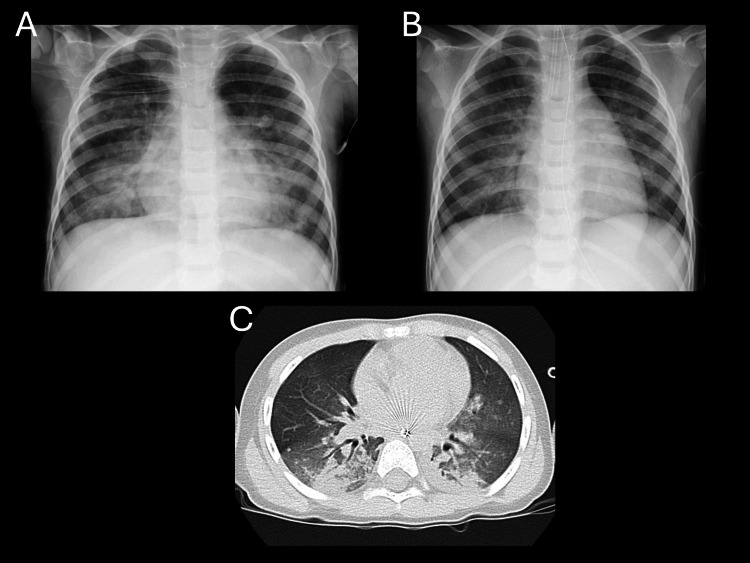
Chest radiographs and chest CT images in case 3 (A) Chest radiograph obtained before prone positioning. (B) Chest radiograph obtained after prone positioning, demonstrating improved radiolucency in the lower lung fields compared with panel A. (C) Chest CT image obtained before prone positioning, showing dorsal atelectasis. Note: The left side of each image corresponds to the patient’s right side.
